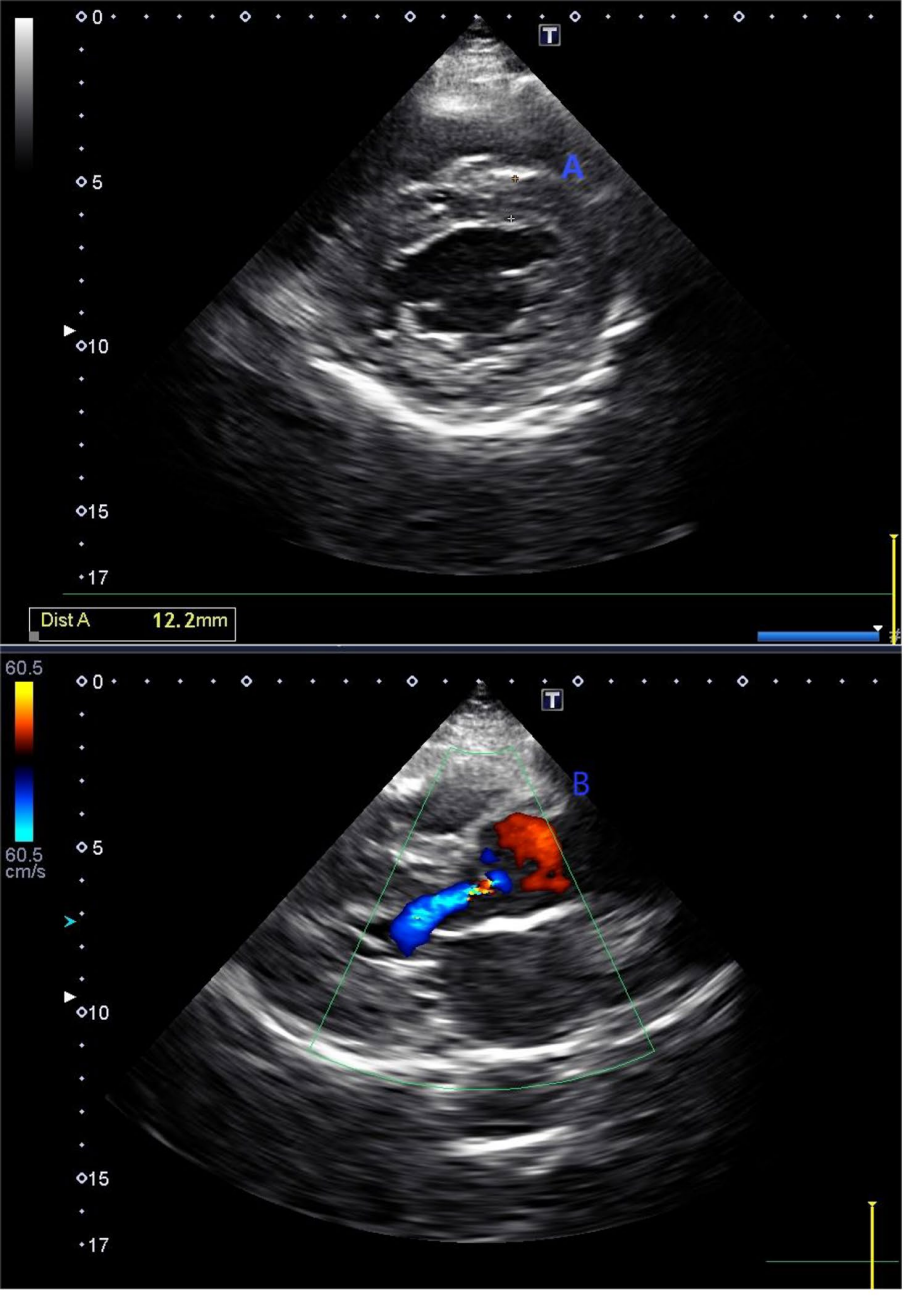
Echocardiography findings. Echocardiography revealed left ventricle wall thickening (A) and mild aortic valve regurgitation (B). The ascending aorta diameter was 30 mm, left atrium anteroposterior diameter was 34 mm, interventricular septum thickness in diastole was 12 mm, left ventricular end-diastolic dimension was 47 mm, left ventricular ejection fraction was 68%, left ventricular posterior wall thickness in diastole was 11 mm, left ventricular end-systolic dimension was 29 mm, main pulmonary artery was 21 mm, E was 0.85 m/s, A was 0.57 m/s, and E/A was 1.51
